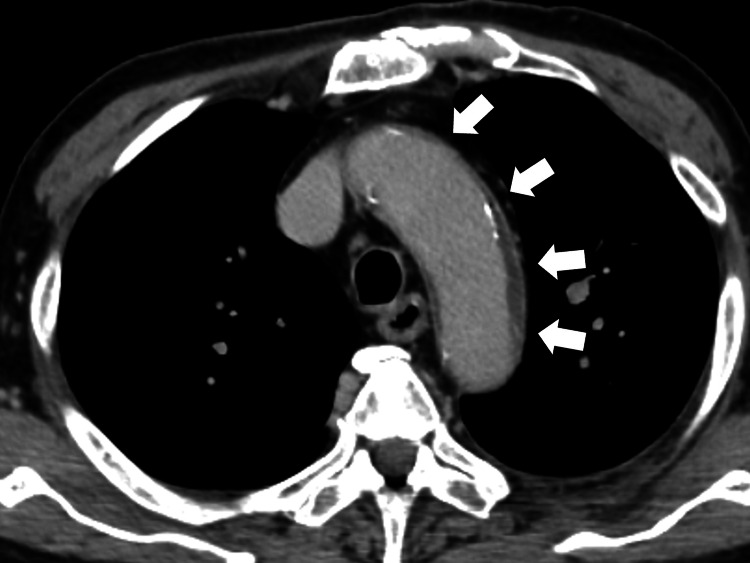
Chest-to-pelvic contrast-enhanced computed tomography showing wall thickening of the aortic arch (white arrows).
